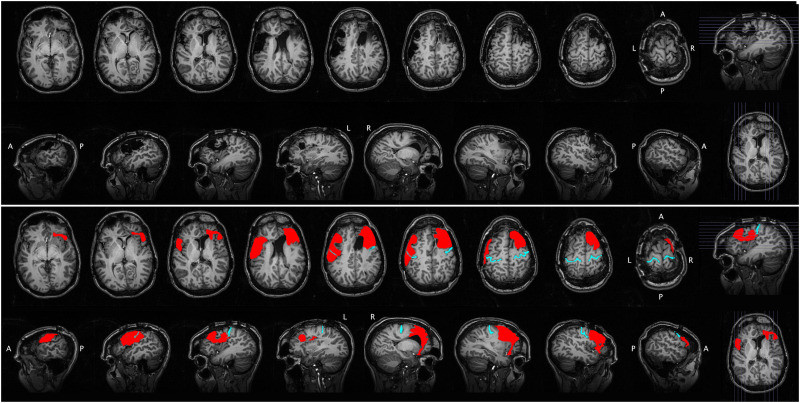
Structural MRI for Case 1. The raw scan is shown in the top portion of the figure, with axial slices (first row) and sagittal slices (second row). The same scan is shown in the bottom portion of the figure, with the lesion segmentation highlighted in red and the central sulcus highlighted in cyan for reference. A = anterior, P = posterior, L = left, R = right.
